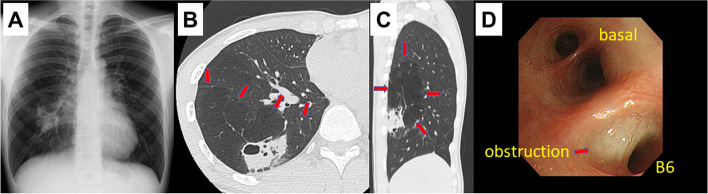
A Initial chest radiograph showed an abnormal shadow in the right lower lung field. B, C Chest CT scan revealed an abnormal mass with an emphysematous change. Arrows show the emphysematous region. D Obstruction of the bronchi was found by bronchofiberscopy
